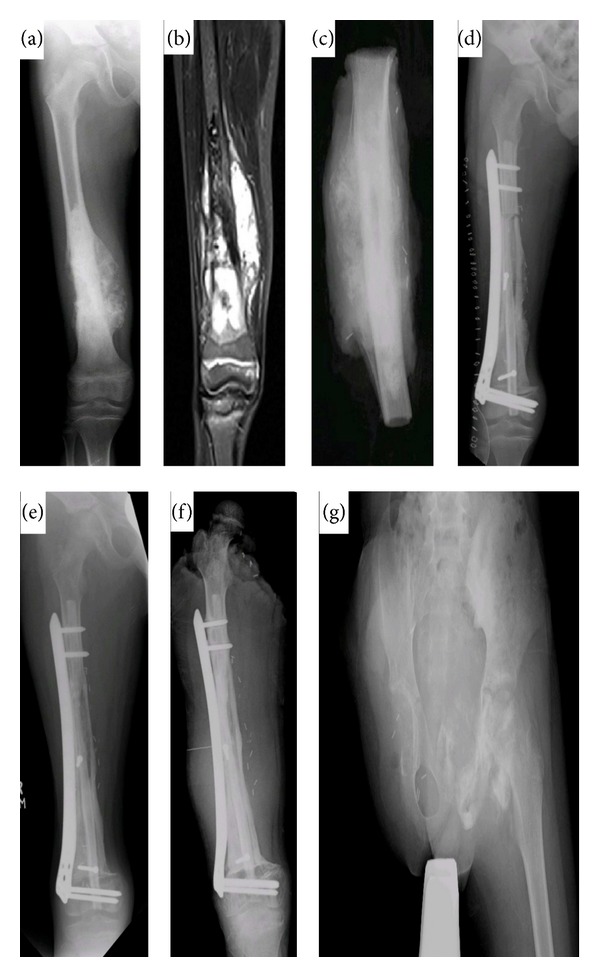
A 10-year-old boy with osteogenic sarcoma. (a) X-ray shows lytic mass in right distal femur. (b) MRI shows osteoid lesion in the metadiaphyseal region of right distal femur. (c) X-ray of the resected bone (d) Immediate postoperative X-ray. (e) X-ray shows graft and host union at both proximal and distal ends 10 months post-operatively. (f) 3-year postoperative X-ray shows local recurrence. (g) X-ray after disarticulation of right hip.
